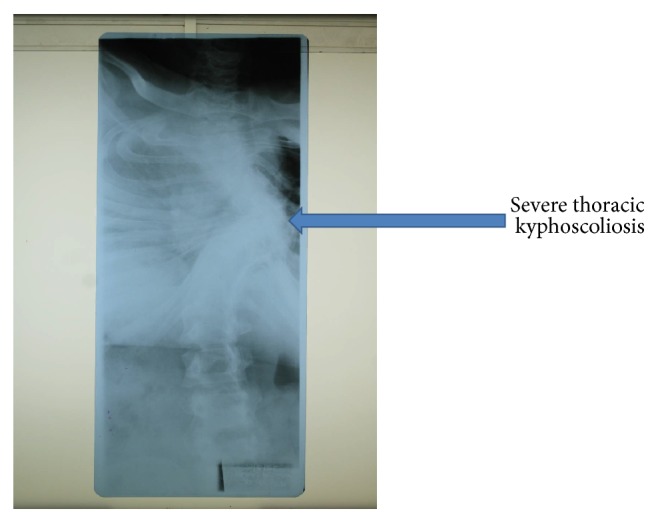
Chest X-ray showed severe kyphoscoliosis in a 25-year-old male with Prader-Willi Syndrome.
